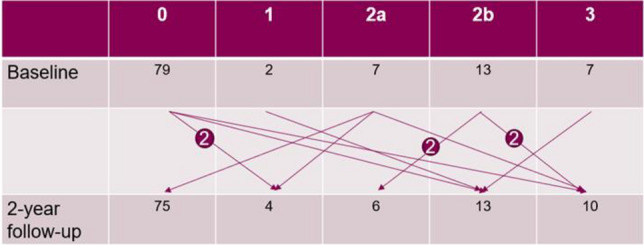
Change in grading of temporomandibular joint deformity (0, 1, 2a, 2b, 3) evaluated with cone beam computed tomography. Data presented on the joint level, an arrow representing one joint unless specified with a number.
